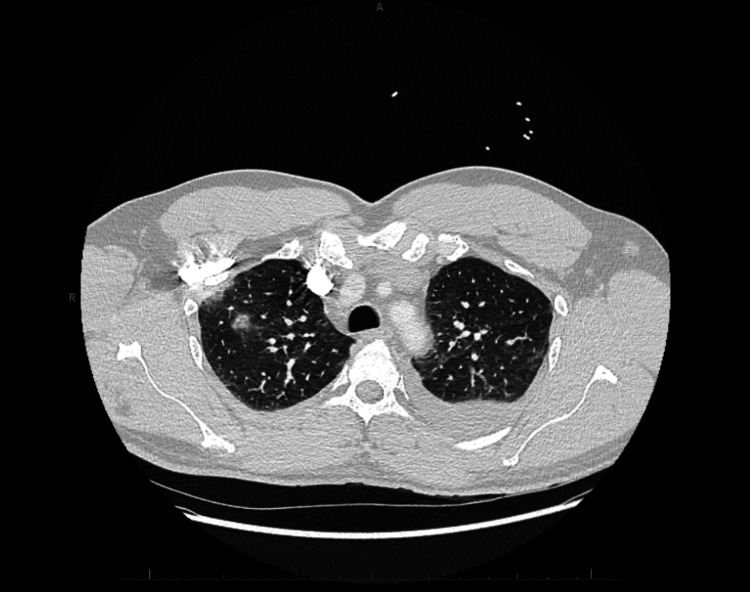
Axial CT of the chest shows bilateral pleural effusions with consolidations and ground-glass opacities, indicating pneumonia on day 6
